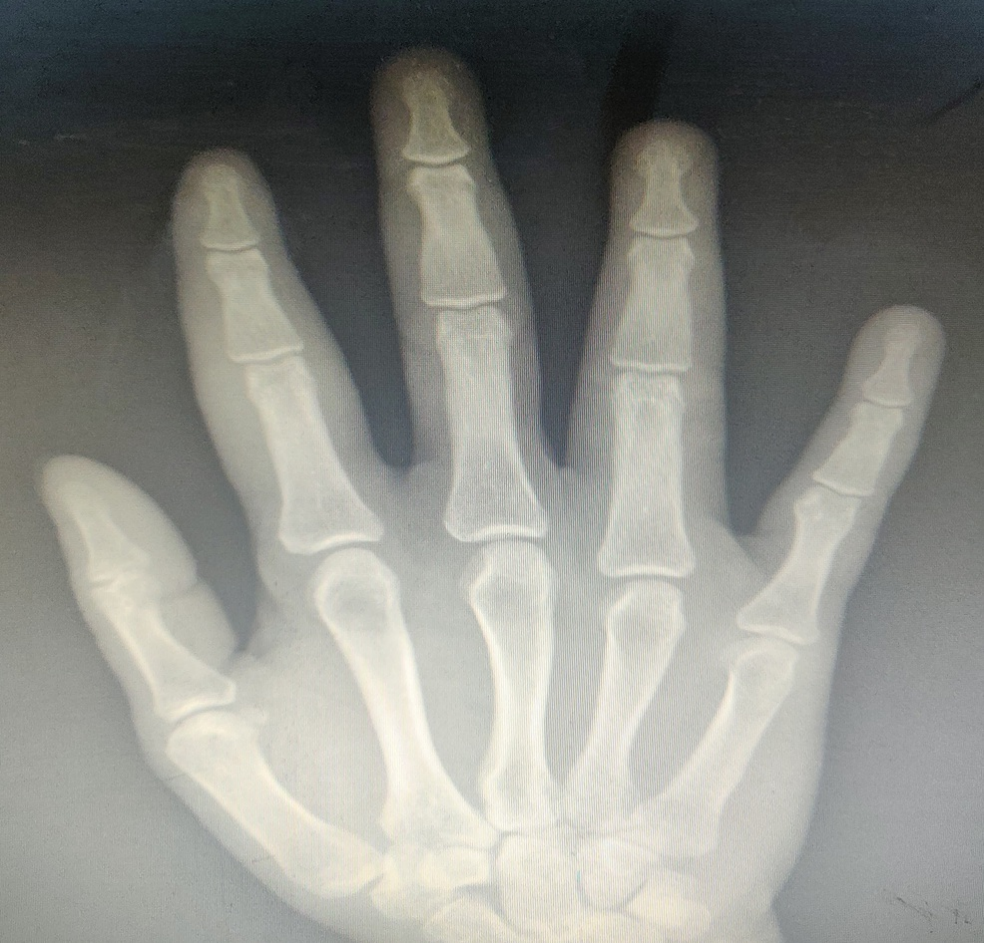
Hand X-Ray depicting mild edema of the soft tissue of the right palm, as well as right index and ring fingers
